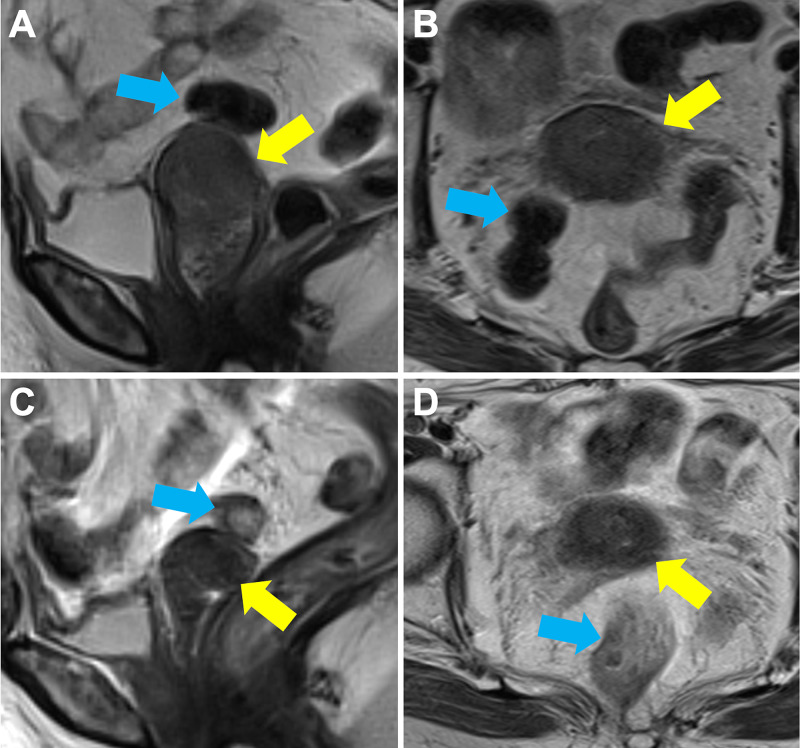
T2-weighted magnetic resonance images of the cervical stump tumor. (A) Time of diagnosis, sagittal plane. (B) Time of diagnosis, axial plane. (C) Before initiation of brachytherapy, sagittal plane. (D) Before initiation of brachytherapy, axial plane. Yellow arrows indicate the primary tumor. Blue arrows indicate the rectosigmoid colon located in close proximity to the tumor.
